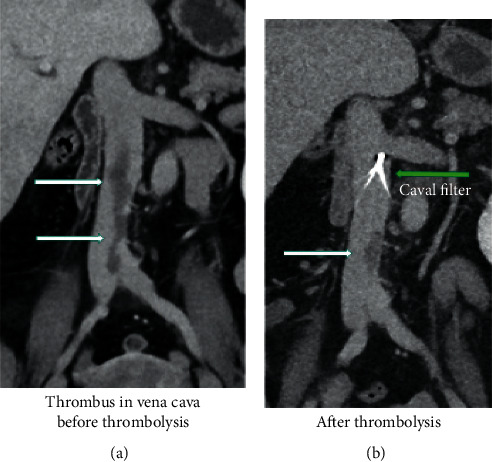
CTPA demonstrating a thrombus of 11 centimetre in the inferior vena cava. (a) The thrombus in the IVC before thrombolysis (white arrows); (b) the residual thrombus (white arrow) following thrombolysis and after insertion of the vena cava filter (green arrow). Abbreviations: CTPA: computed tomography pulmonary angiogram, IVC: inferior vena cava.
